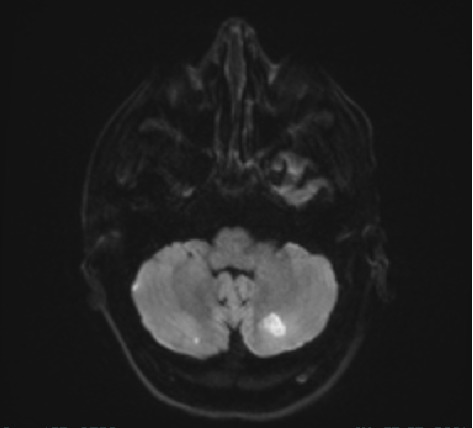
Brain MRI showing multiple area of infracts involving bilateral cerebellum and right frontal.
